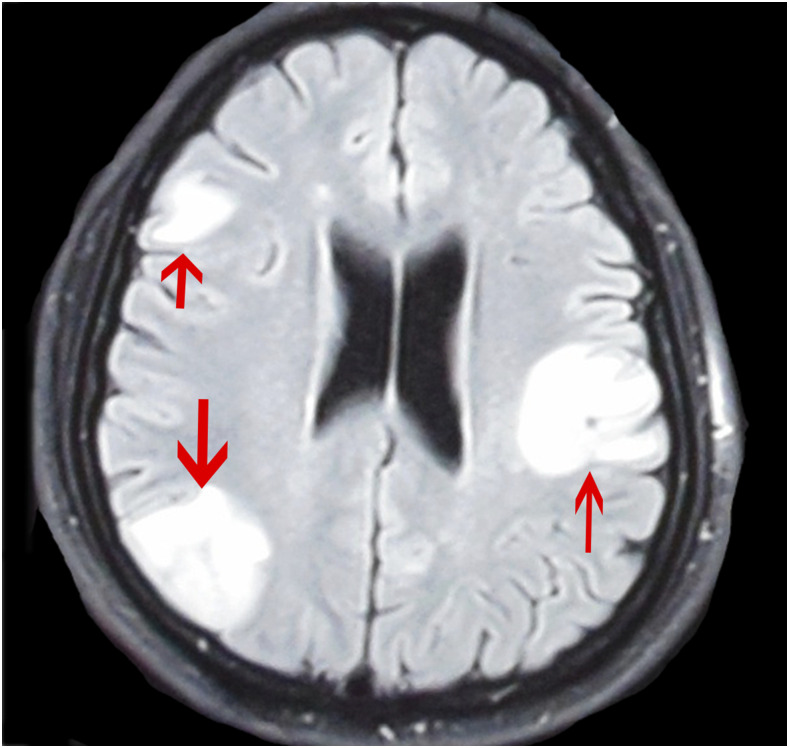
Persistent edema on imaging studies. A 6-month posttreatment axial T2-FLAIR MRI scan from a patient with neurocysticercosis. The scan shows persistent edema in multiple areas, indicated by arrows. This figure appears in color at www.ajtmh.org.
